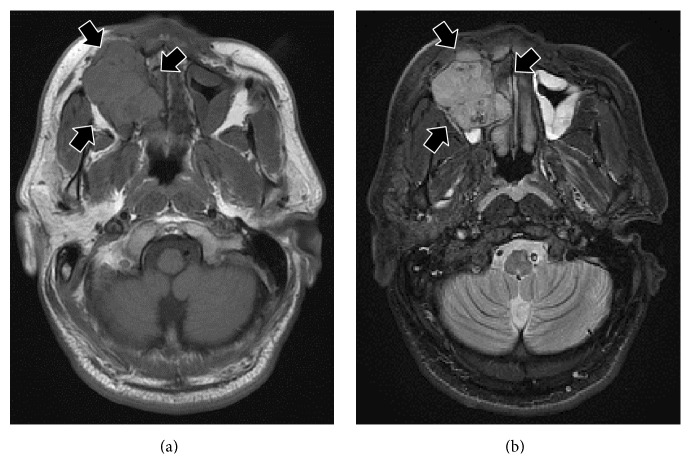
An MRI scan shows a mass with low T1- (a) and moderate T2-weighted (b) signal intensity.
